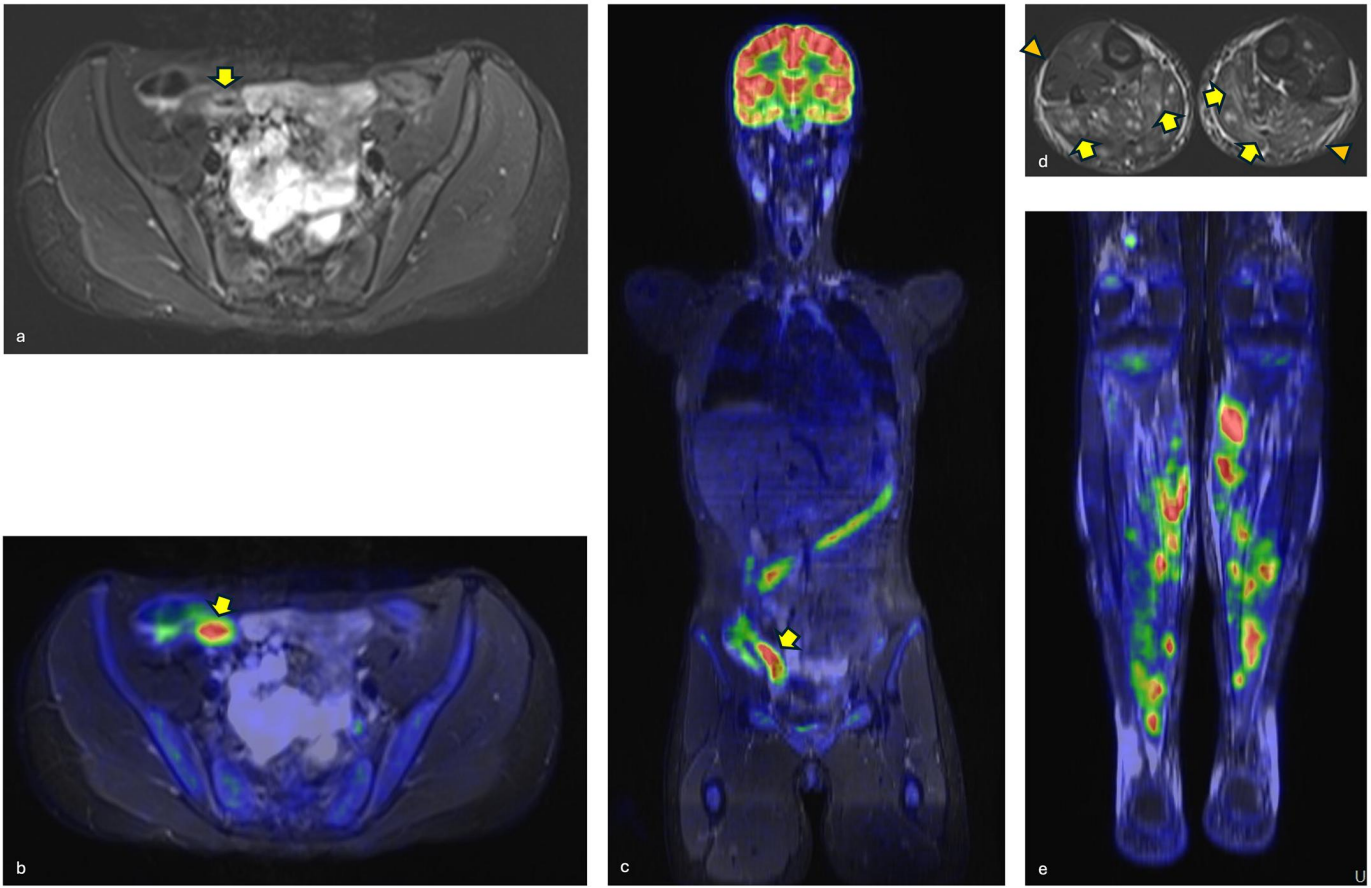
Whole-body [18F]-FDG-PET/MR showing (a) the thickened terminal ileal loop (yellow arrow), (b) the increased FGD uptake on the fused STIR and PET imaging (yellow arrow) and (c) on the coronal view. Diffuse tracer uptake, corresponding to the muscle and fascial edema of the calves (yellow arrow and orange arrowheads, respectively), is evident in (d) the axial STIR and (e) coronal fused STIR/PET imaging.
